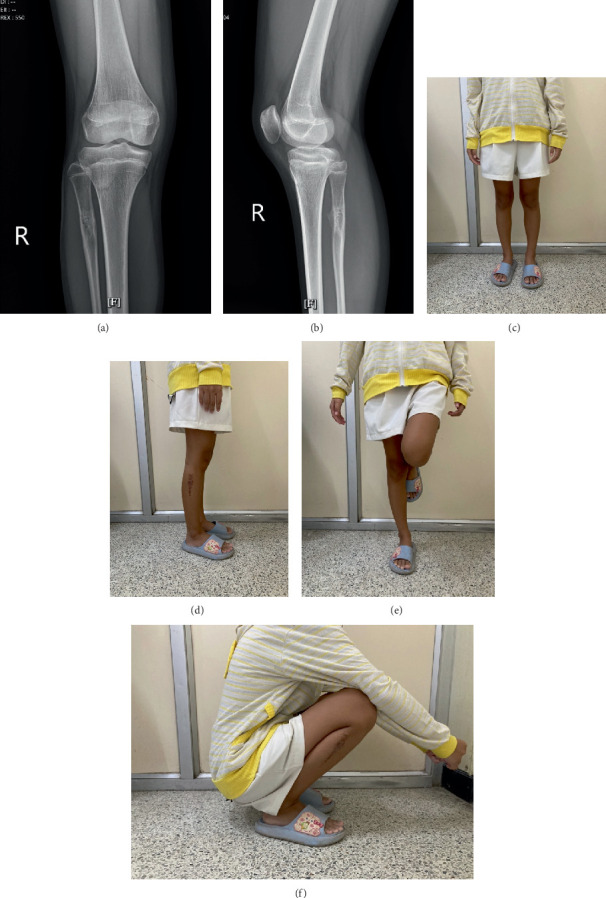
At 18 months postoperation. (a, b) Plain radiographs show excellent remodeling of the allograft. (c–f) Excellent functional outcomes with an MSTS score of 100%.
